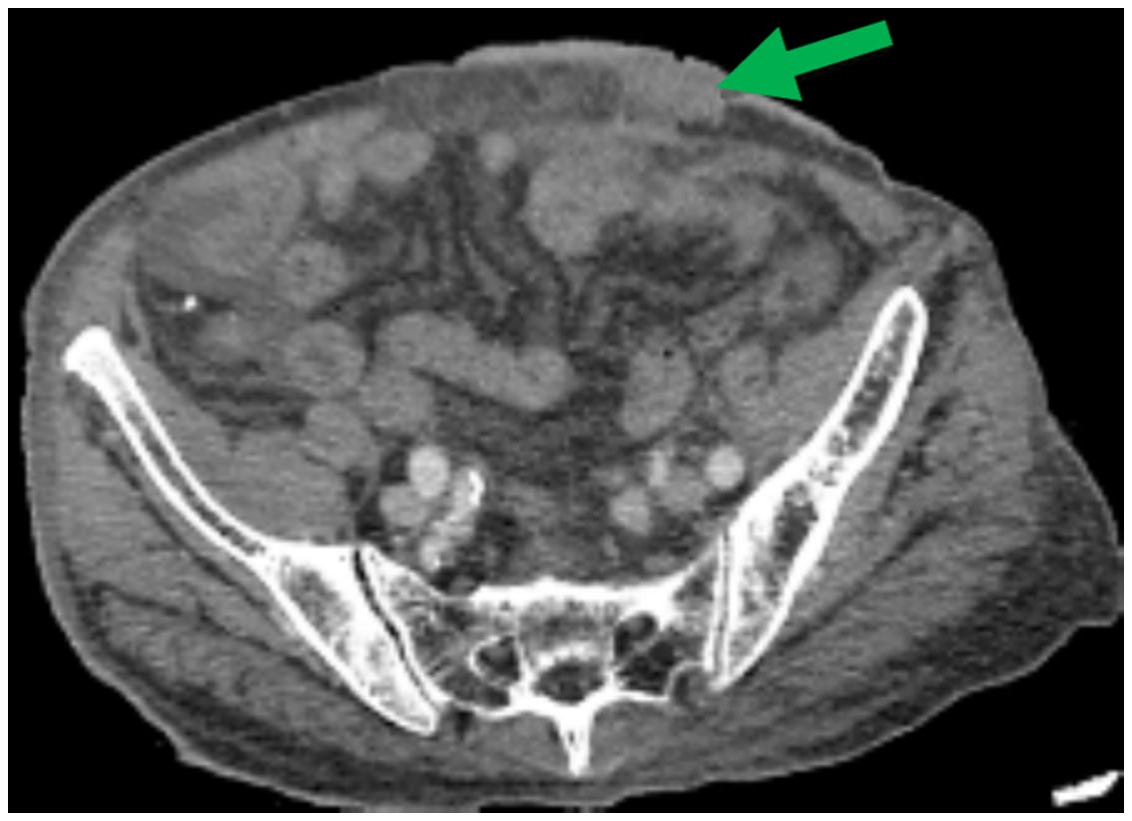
Postoperative enhanced abdominal CT revealed a significant disappearance of most varicose veins around the stoma (green arrow).
